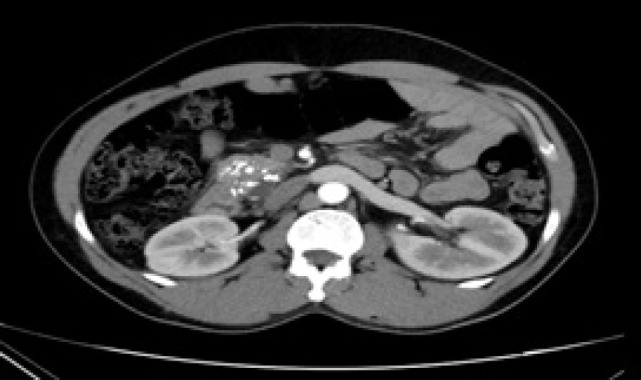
Computerized tomography (CT) scan after 3-year follow-up showing almost complete resolution of the mid-polar small renal mass in the right kidney.
